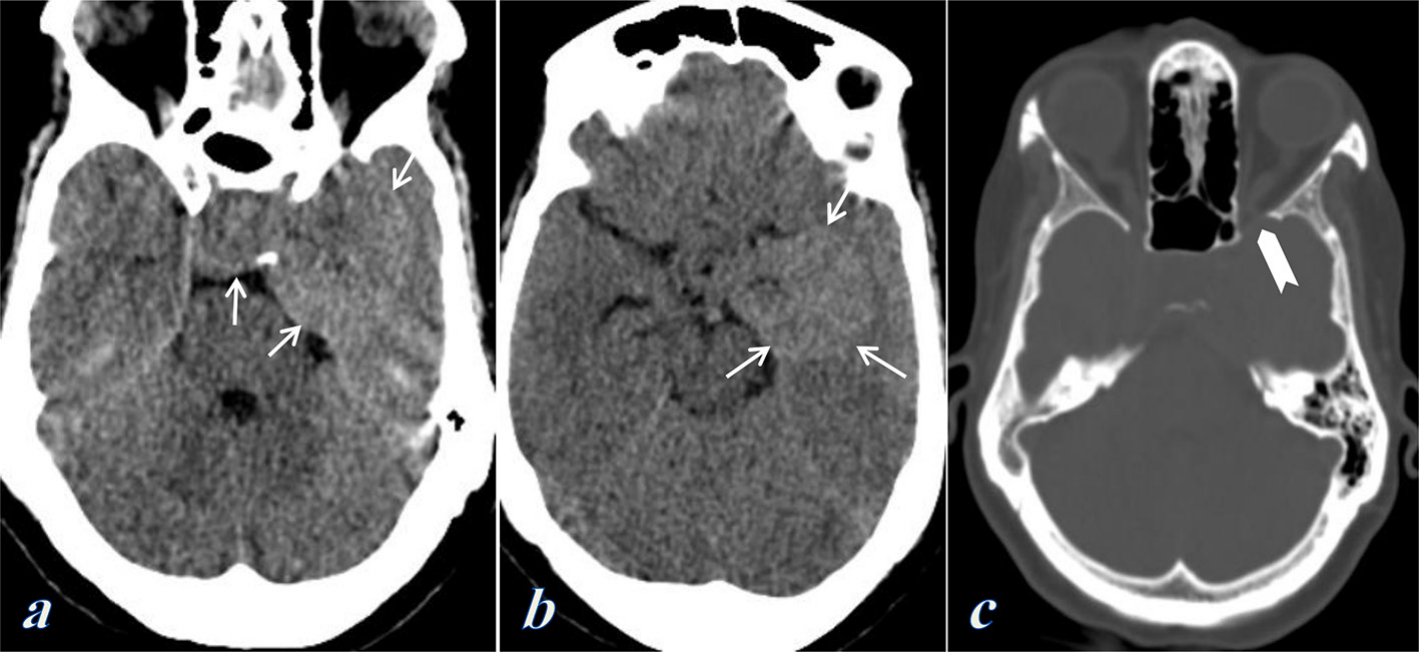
Non-contrast CT scan of the brain; (a, b) Axial soft tissue window showed a large extra axial sellar and left parasellar mass which is isodense/slightly hyperdense to the brain parenchyma (Arrows). It effaces the prepontine cistern, (c) Axial bone window showed bony scalloping and erosion of the medial part of the sphenoid wing (Chevron).
